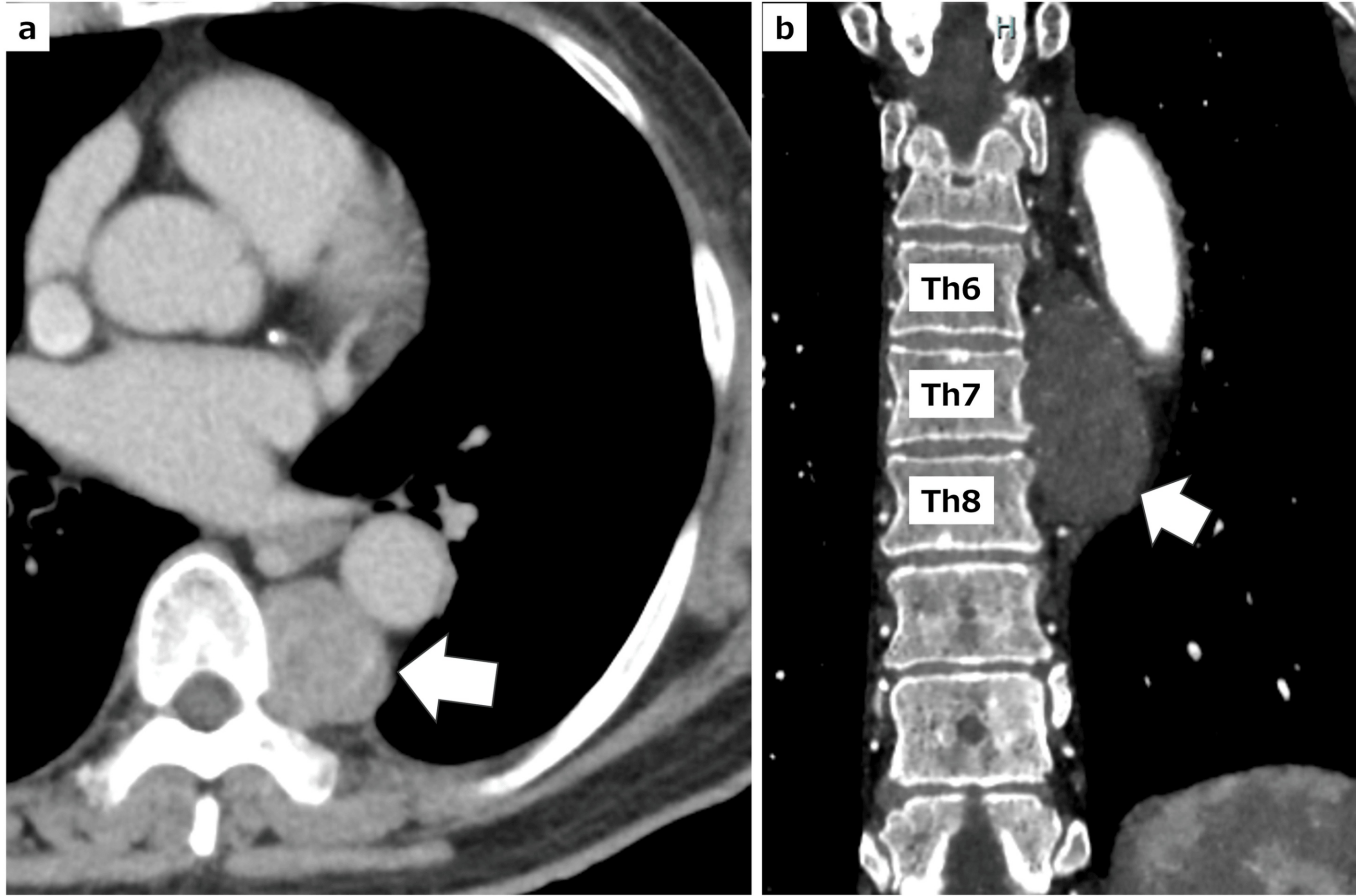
Chest CT findings of the posterior mediastinal tumor a: Axial CT image showing the tumor located between the vertebral bodies and the aorta (white arrow). b: Coronal CT image showing the tumor situated on the left side of the sixth to eighth thoracic vertebrae and adjacent to the descending aorta (white arrow). CT: computed tomography
